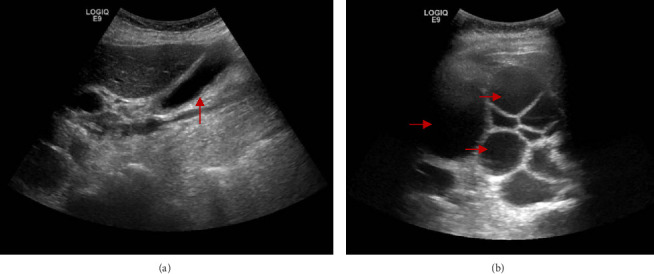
Hepatobiliary ultrasonogram showing the gallbladder (red arrow (a)) and right lobe of the liver (red arrows (b)).
